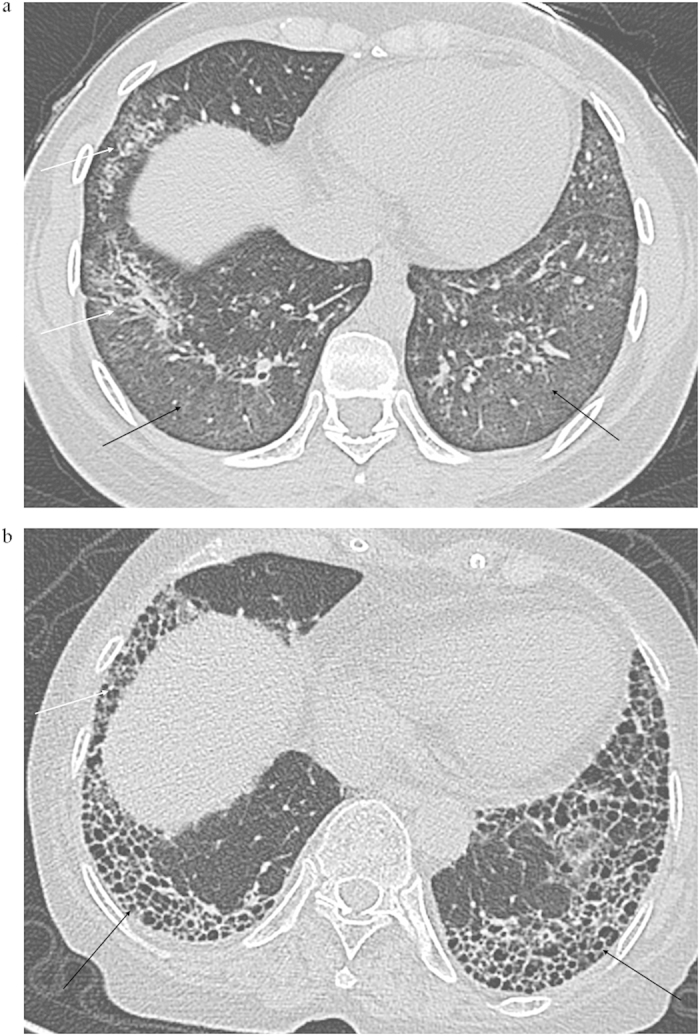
Representative HRCT images for RA-ILD. (a) ground-glass opacification (black arrow) and traction bronchiectasis (white arrow), (b) Honeycombing (black arrow) and reticular abnormalities (white arrow).
